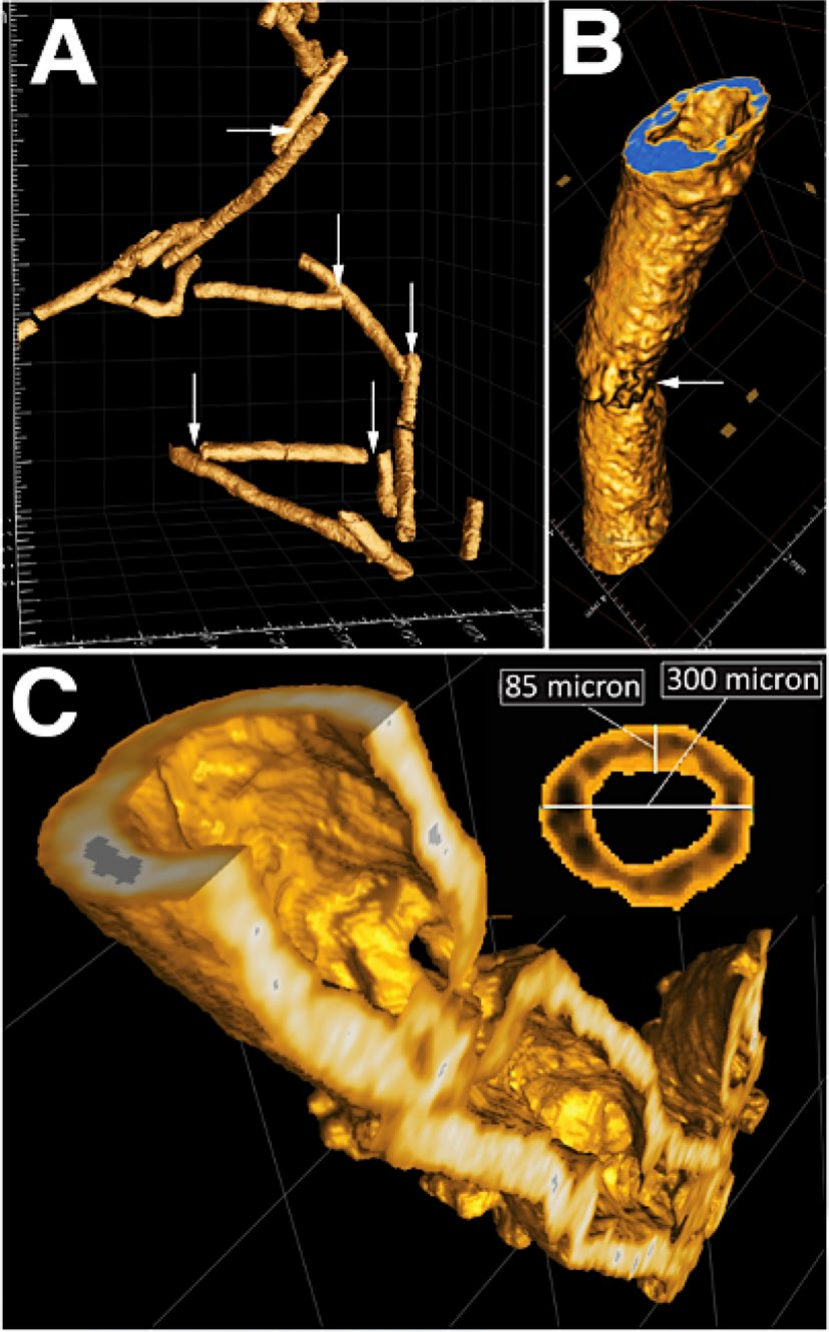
(A) Shows a filtered micro CT sub-volume highlighting the geometry and morphology of TPS (PTs were excluded this reconstruction). Note that TPS exhibit sheath-like morphology with collapse fabric that was most likely acquired during dewatering and bed compaction. The presence of well-preserved “knick points” (white arrows) indicates that TPS were constructed relatively early, prior to significant mud compaction. (B) close-up of a representative TPS. Note the well-developed knick separating two well-developed pyritic tubular bodies. (C) shows a vertical cross section through a representative TPS. Note the well-developed and continuous sheath morphology with a pyritic wall thickness of ~ 85 micron and a continuous overall diametre of ~ 300 micron.
